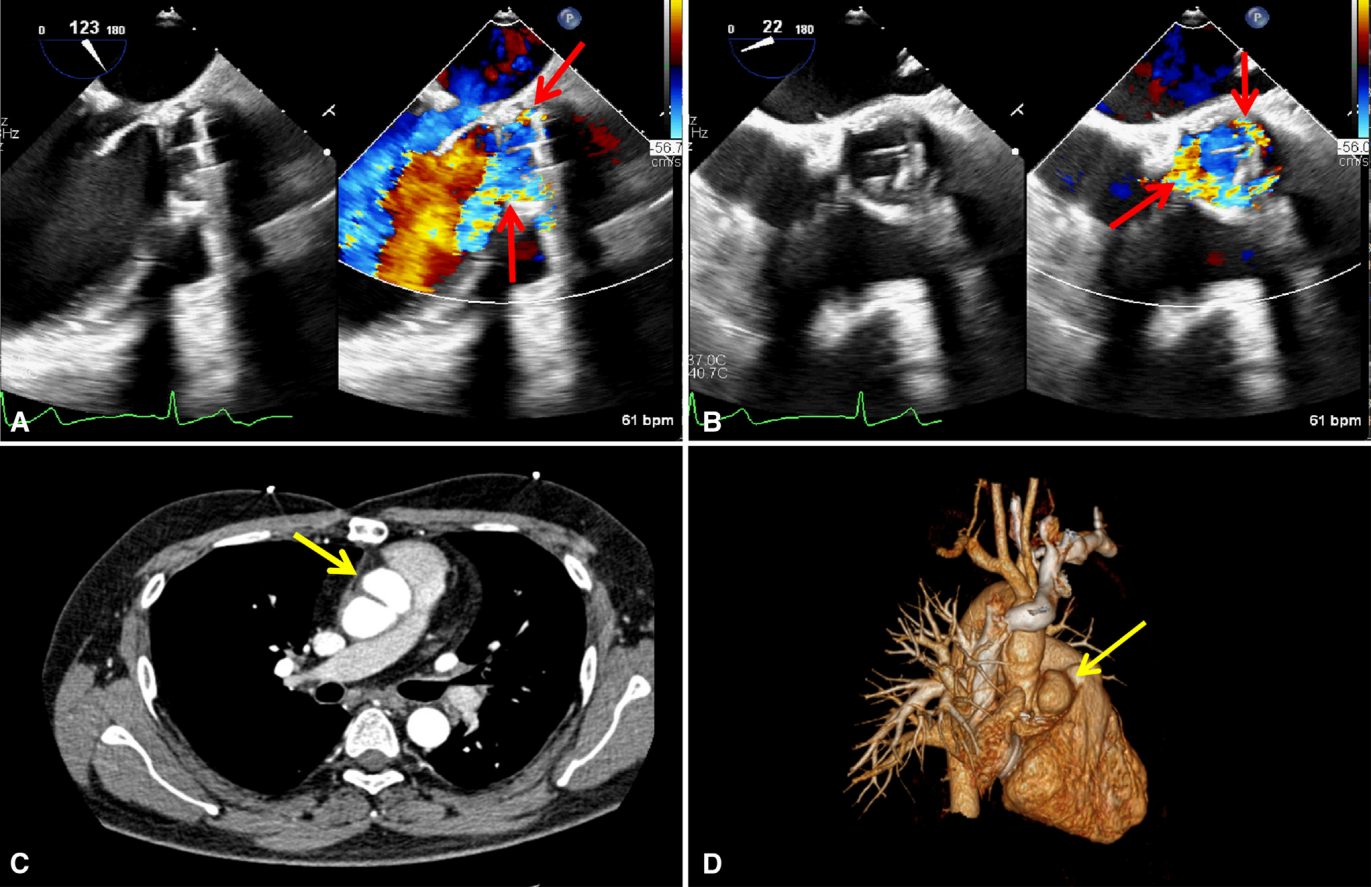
A and B, Preoperative transesophageal echocardiogram demonstrating severe perivalvular regurgitation (*red arrows*). C and D, Computed tomography angiography showing a pseudoaneurysm originating the aortic root (*yellow arrows*).
